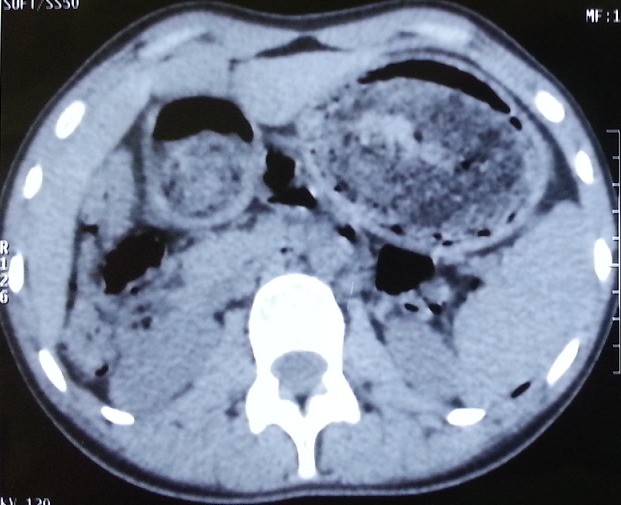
Preoperative CT scan: coronal image of the gastro-duodenal trichobezoar
